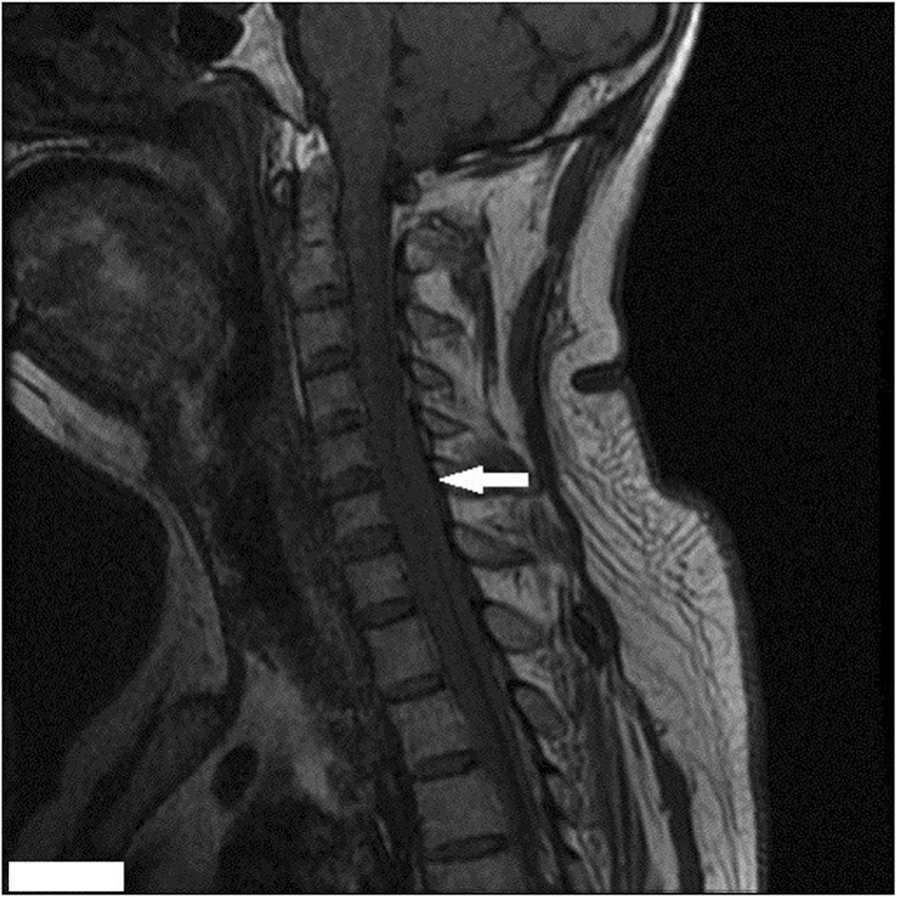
Sagittal MRI of the cervical spine (T1-weighted image). Hypointense collection over the posterior aspect of the spinal cord with cord edema can be seen
